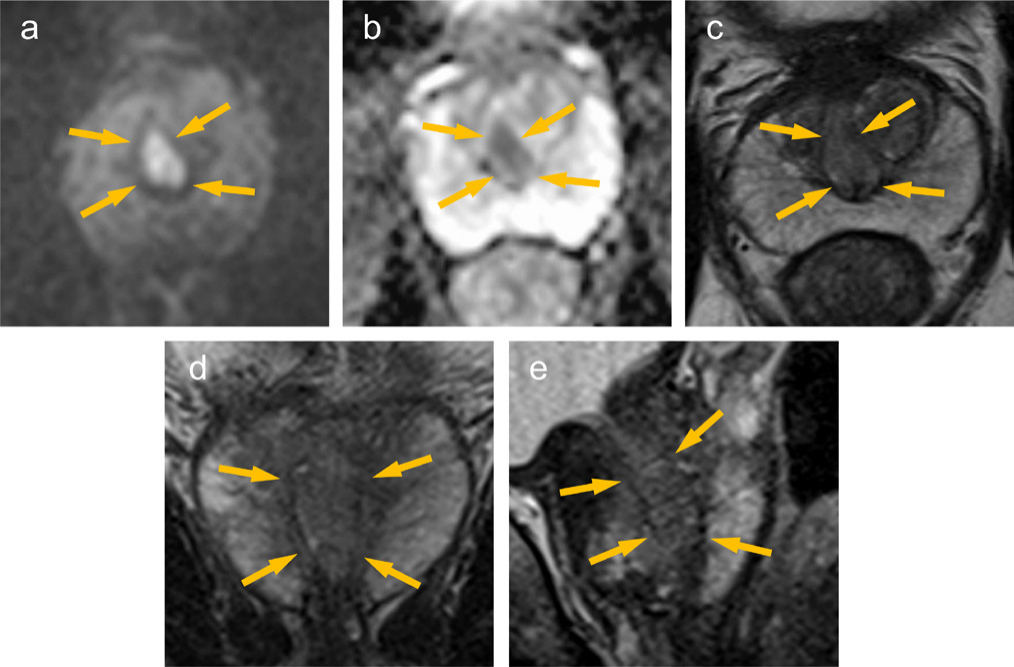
MRI (3.0T). There is an occupying lesion in the prostatic urethra (→). The lesion shows hyperintense signal on DWI (a), hypointense signal on ADCmap (b). On T2WI, the lesion shows slightly inhomogeneous hypointense signal similar to hypertrophic nodule in the transition zone (c; axial image, d; coronal image, e; sagittal image).
